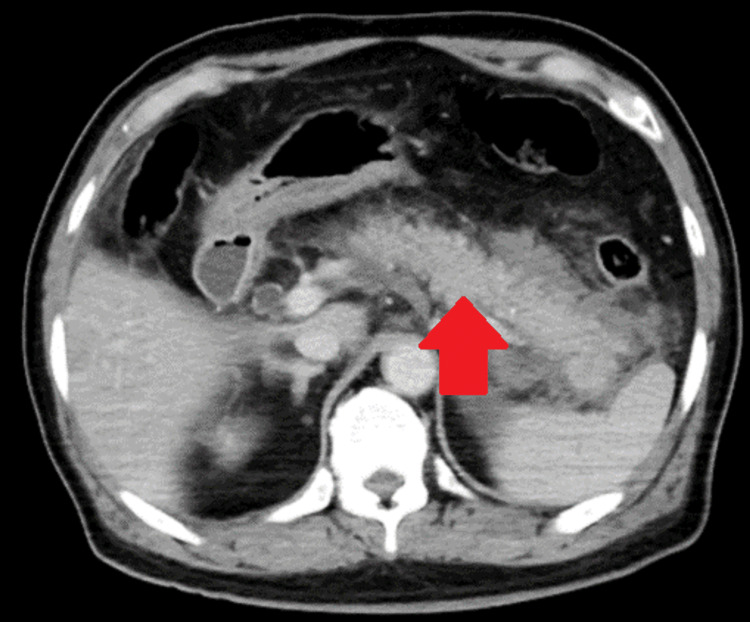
Contrast-enhanced abdominal CT indicating pancreatic parenchyma and the surrounding inflammation (red arrows)
